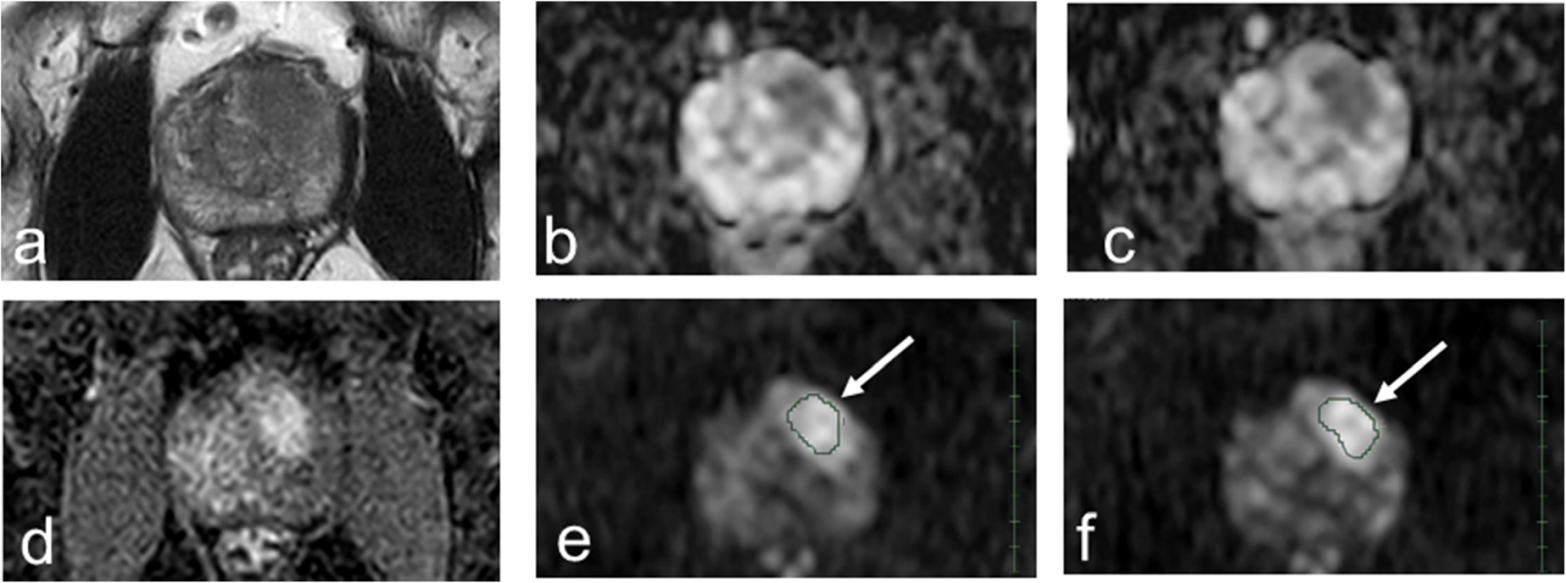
Multiparametric magnetic resonance imaging of the case (61 years, transition zone cancer, GG of 2, PI-RADS of 5, T2cN0M0). a Axial T2-weighted image (repetition time of 4,000 ms and echo time of 80 ms). b First axial apparent diffusion coefficient (ADC) map (100, 1,000). c Second axial ADC map (100, 1,000). d Dynamic contrast-enhanced T1-weighted image. e First DWI 1,500. f Second DWI 1,500. Arrows indicate polygonal areas of the region of interests on e and f
